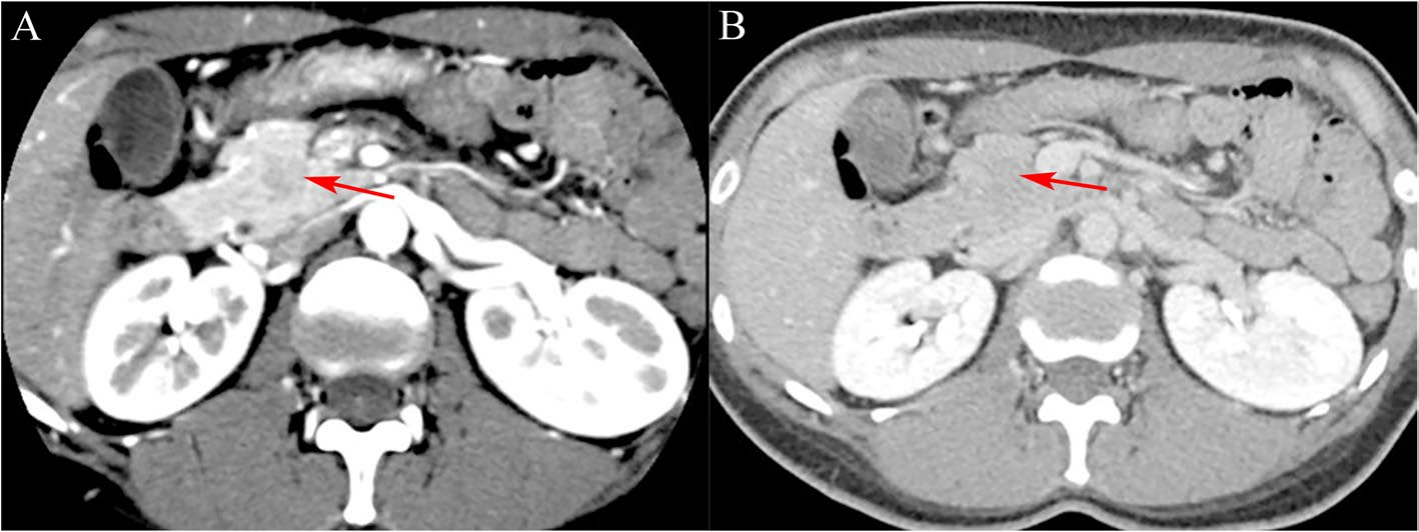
A 25-year-old male with a solid pseudopapillary tumor. CT target scanning shows that (a) the SPTP is located on the pancreatic head, and the capsule is complete and enhanced linearly (long arrow) during the pancreatic parenchymal phase; (b) the tumor is enhanced to the same degree as the pancreas and shows isoenhancement during the delayed phase (long arrow)
